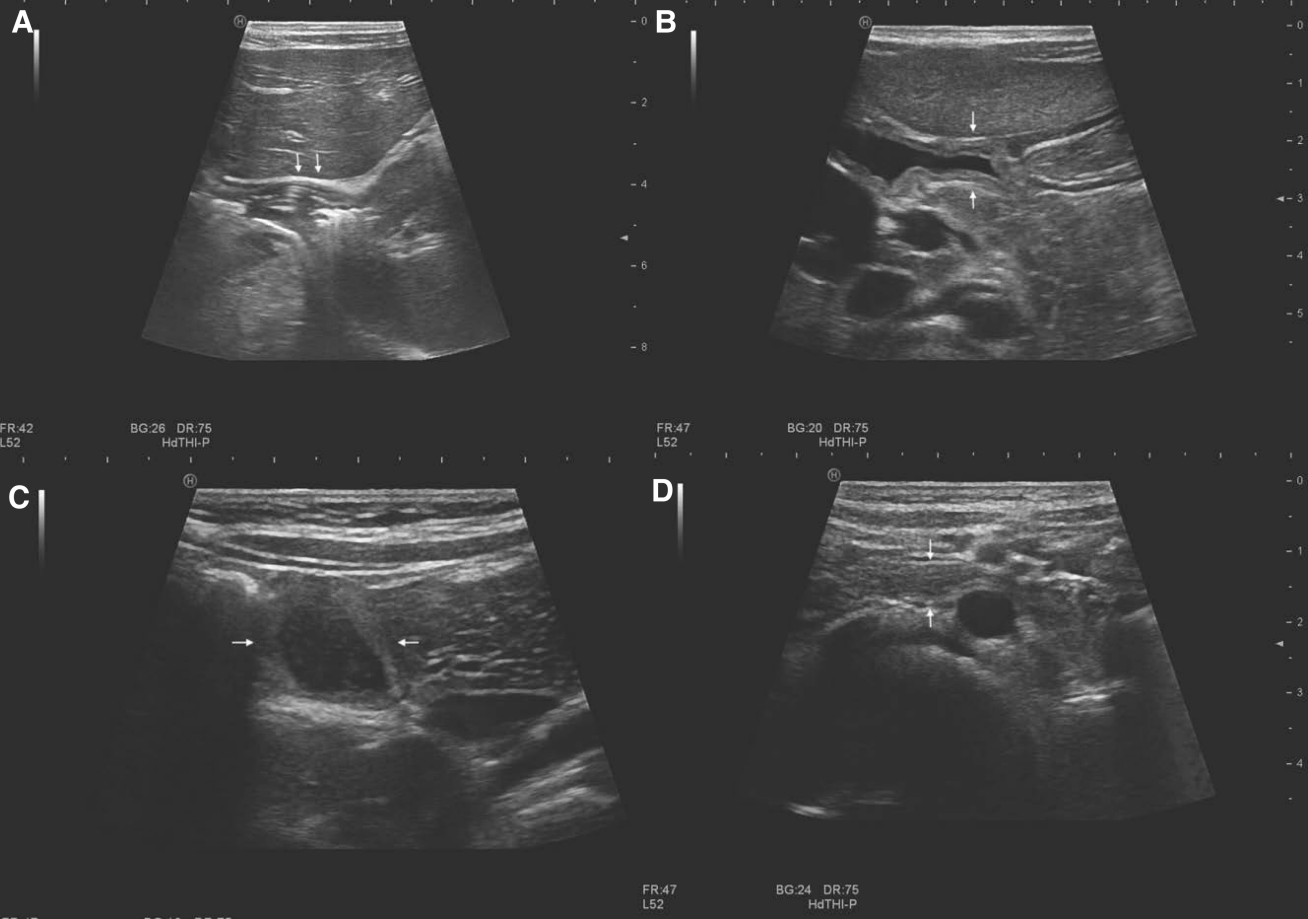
Normal gastrointestinal sonographic images obtained with high-frequency probes in a 2-year-old girl without magnets. Sonograms display the cardia (arrow in A), duodenal bulb (arrow in B), descending portion of duodenum (arrow in C), and horizontal portion of the duodenum (arrow in D).
